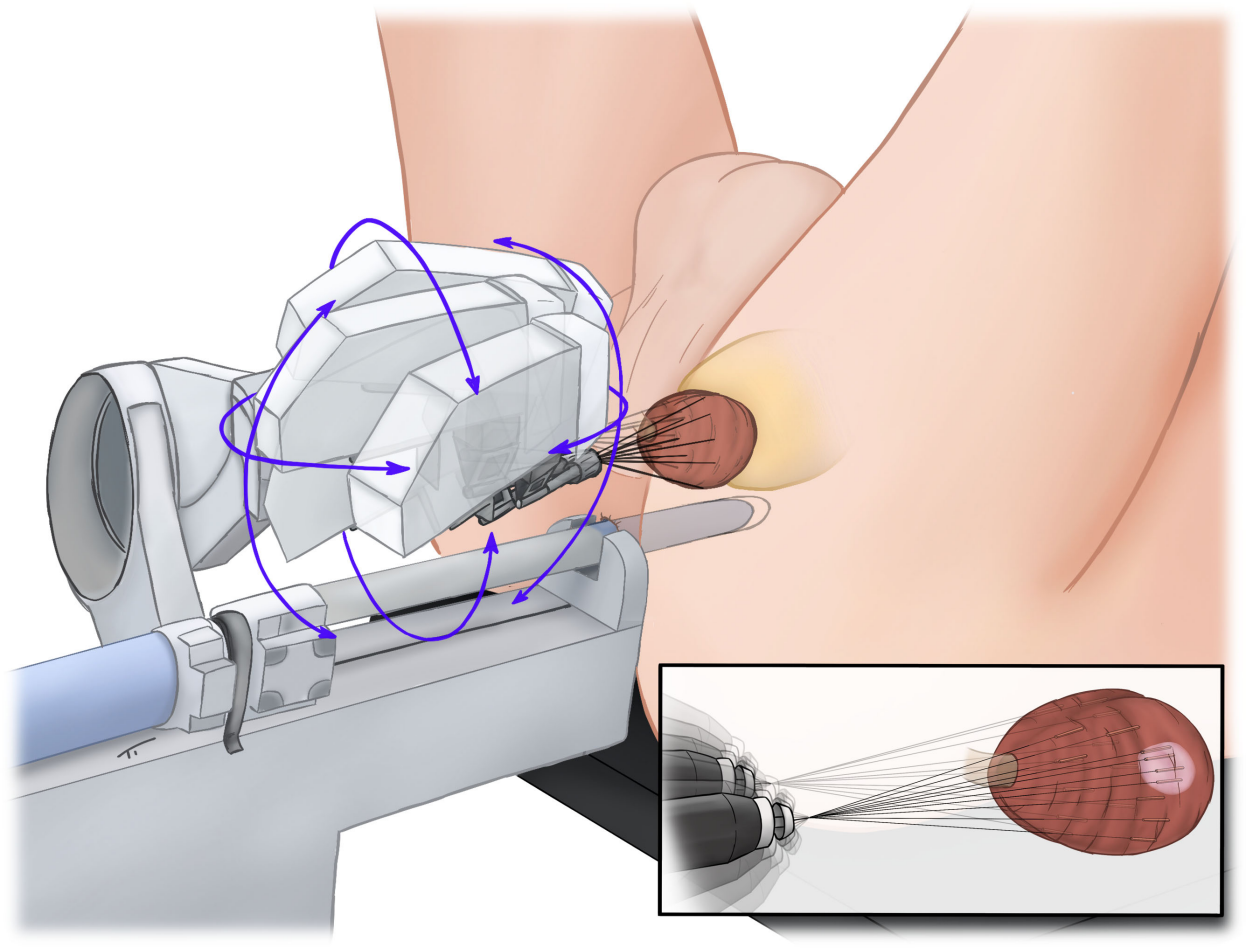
Robotic-assisted transperineal MRI-TRUS-fusion guided biopsy of the prostate.
